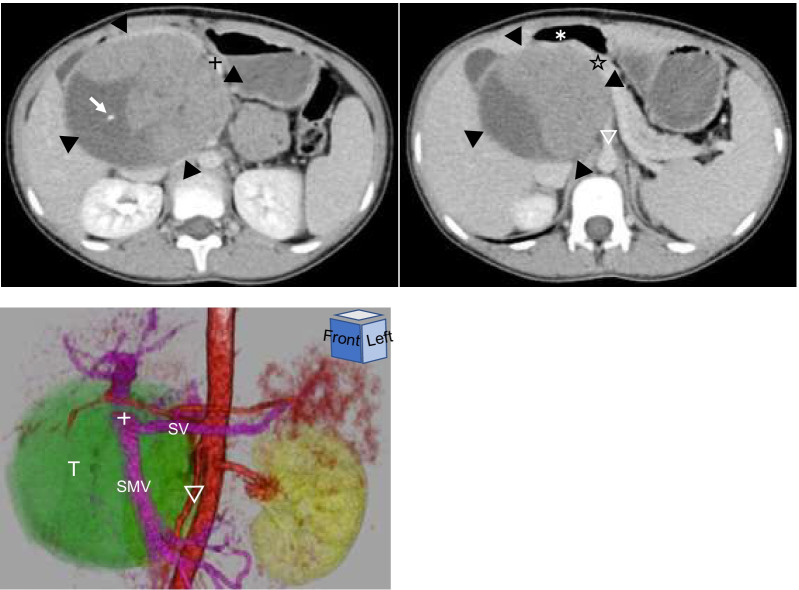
Contrast-enhanced CT scan and 3D imaging on admission. The tumor is located in the retroperitoneum, including the pancreatic head, in such a way that the pancreas (☆) is ventralized, the duodenum (✻) is right ventralized, and the extrahepatic portal vein (+) is left ventralized. The mass is well-defined, smooth, and covered with a thick coat-like structure (surrounded by ▼), with thin septum-like structures with microcalcification (→) and papillary enhancing components within the tumor. In the 3D image from the left anterior view, the extrahepatic portal vein (+) runs ventrally to the left in contact with the tumor surface, and the SMA (▽) runs to the left in contact with the tumor surface. *CT* computed tomography, *SMV* superior mesenteric vein, *SV* splenic vein, *T* tumor
